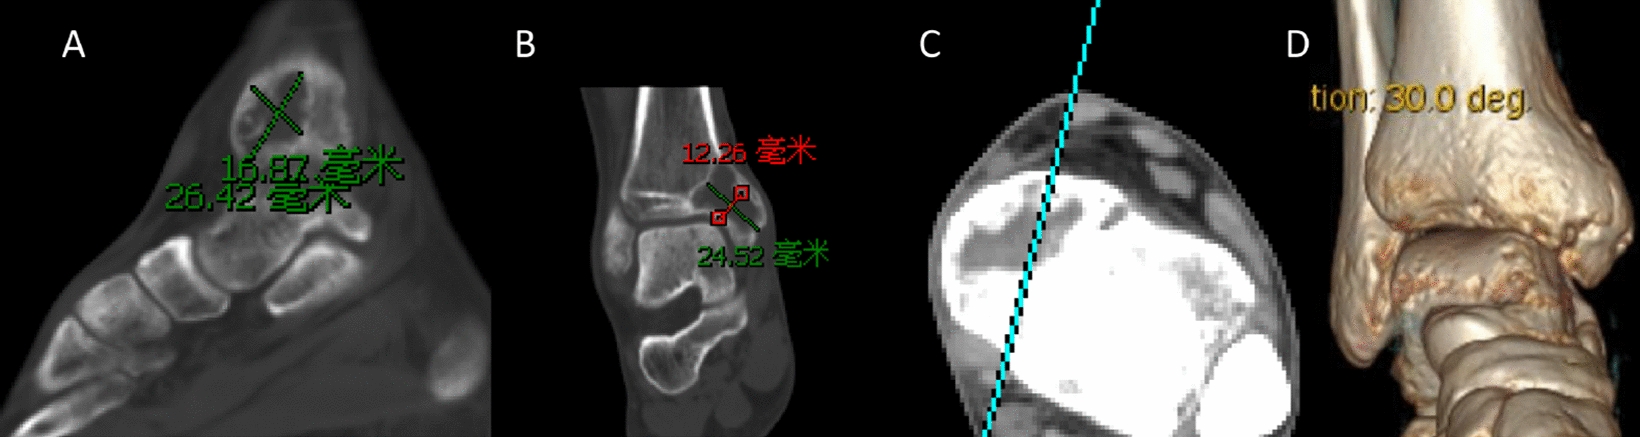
CT results showed that the right tibia distal round cystic bone destruction, expansions, internal trabeculae disruption, bone cortical rupture Swelling of the surrounding soft tissue (a–d)
